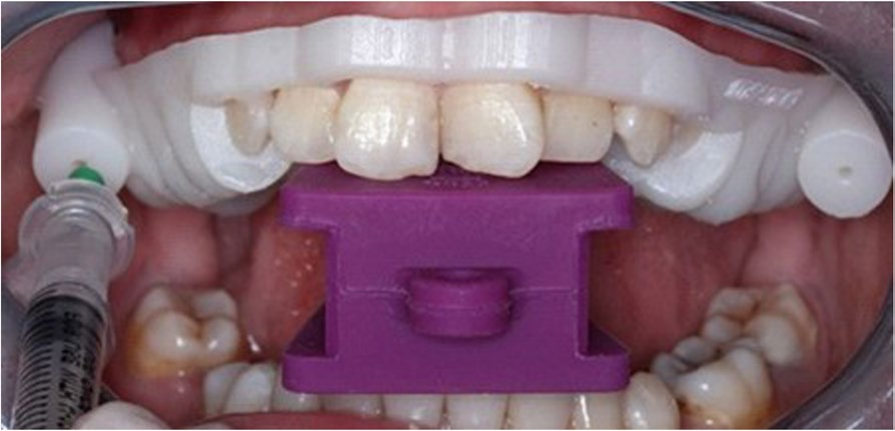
Showing needle insertion guide resting on upper teeth with the same readymade bite block used in CT scan during BTX injection
